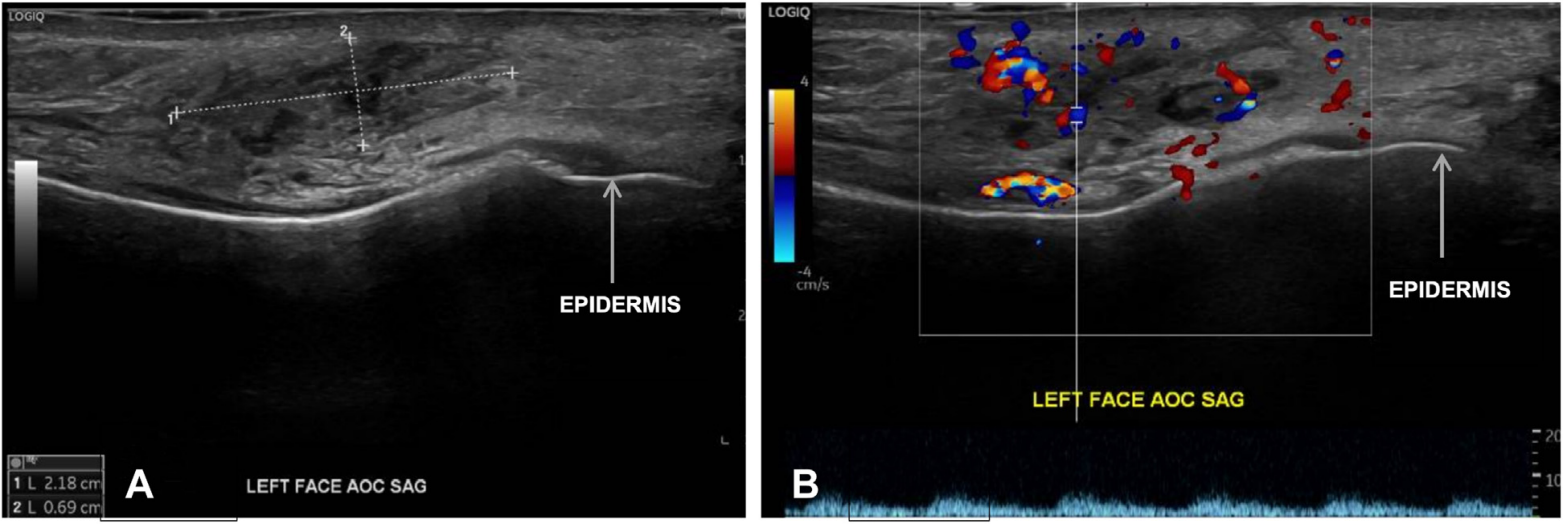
Kimura disease ultrasound. A, Heterogeneous 2.2 × 0.7 cm area of subcutaneous soft tissue thickening on the left face. B, Color Doppler imaging of left face identifying areas of hypervascularity and multiple, normal-sized lymph nodes. *AOC*, Area of concern; *SAG*, sagittal view.
